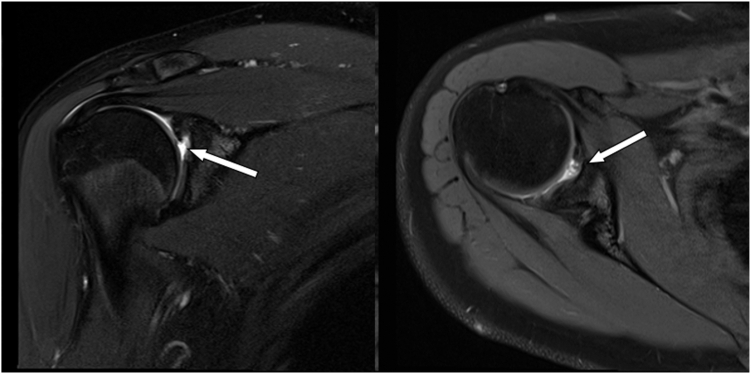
Four months post-microfracture coronal (*left*) and axial (*right*) T2-weighted MRI of right shoulder demonstrating evidence of osteochondral defect (measuring roughly 7 × 9 mm) at the anterosuperior margin of the glenoid. *MRI*, magnetic resonance image.
